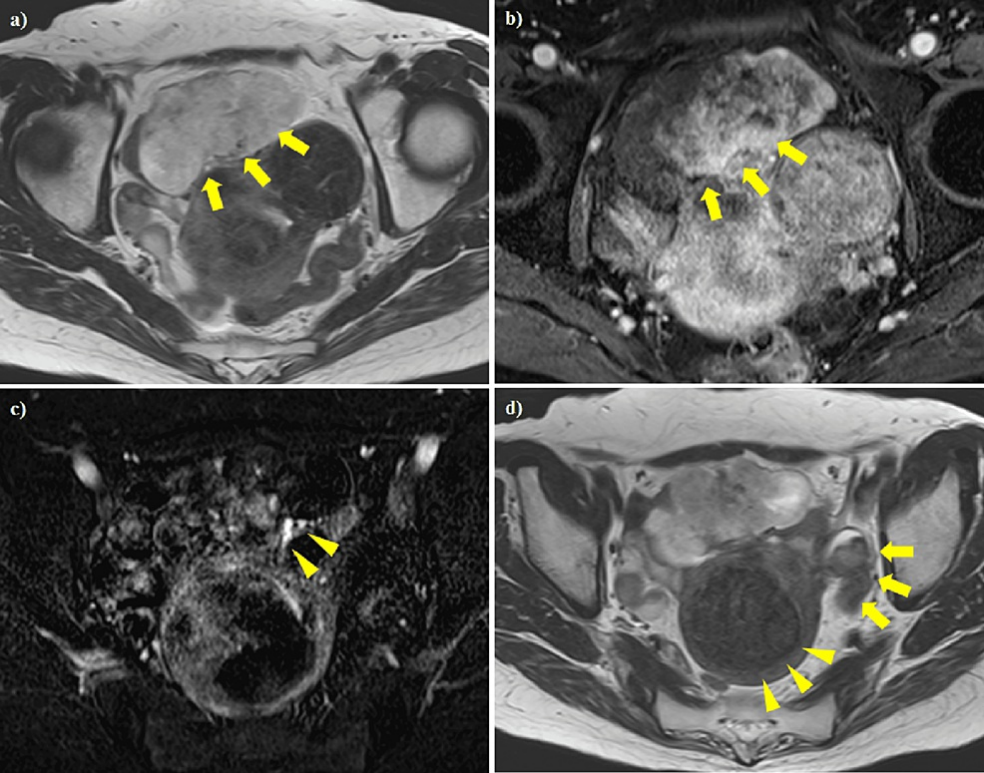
Abdominal pelvic contrast-enhanced MRI. (a) T2-weighted images revealing a heterogeneous lobulated mass. (b) Contrast enhancement. The interior of the tumor showed a progressive contrast effect. There was poor contrast, consistent with a low-signal area in the T2-weighted images. (c) Contrast enhancement. The peripheral branches of the SMV entered the mass. (d) T2-weighted images. The left ovary (arrow) was visible, but the right ovary could not be identified. A uterine myoma (arrowhead) was observed. SMV: Superior mesenteric vein
